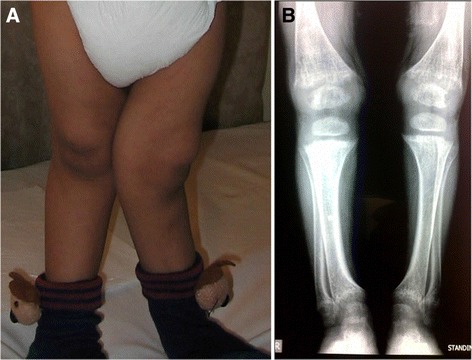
Rickets in cystinosis. a- A cystinosis child with evident rachitic bone deformities. b- Active rachitic bone disease in X-Rays
